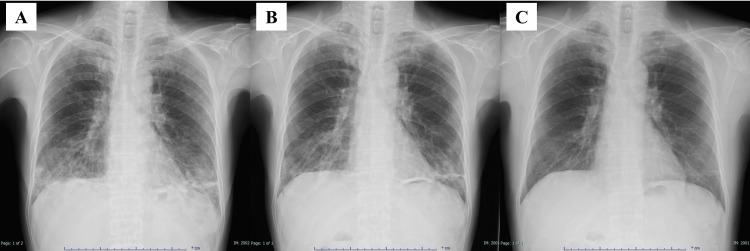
Chest X-ray on admission showing lung infiltration predominantly in the lower lung fields (A), and chest X-ray 62 days (B) and three months (C) after hospitalization showing further improvement.
